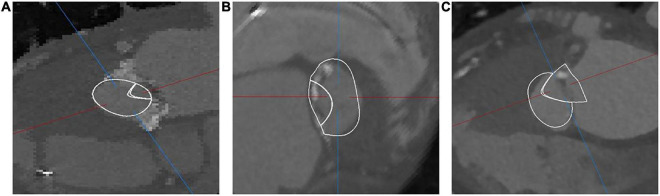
Overlay of computational domain onto CT depicting the shape of the neo-LVOT in VIM-1 (A), VIM-2 (B), and VIM-3 (C).
